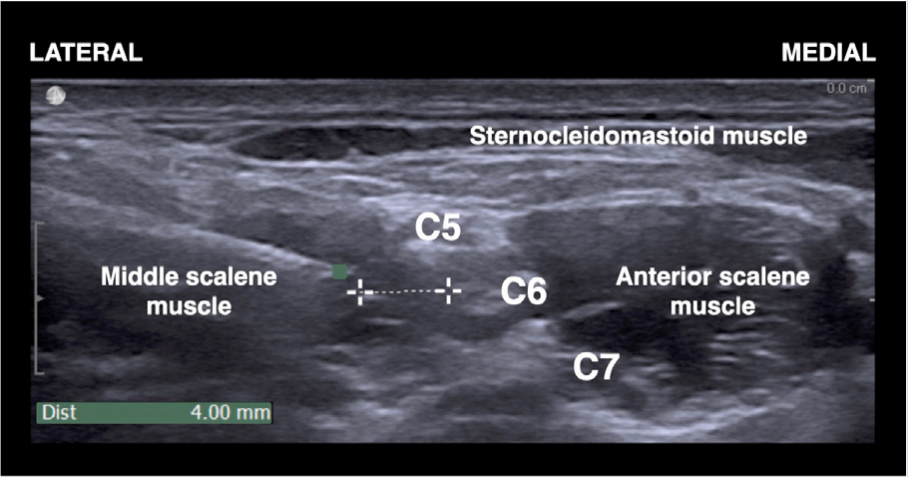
Ultrasound-guided interscalene brachial plexus block with an extrafascial injection: needle tip position at a distance of 4 mm from the lateral border of the brachial plexus sheath; C5, C5 root; C6, C6 root; C7, C7 root.
